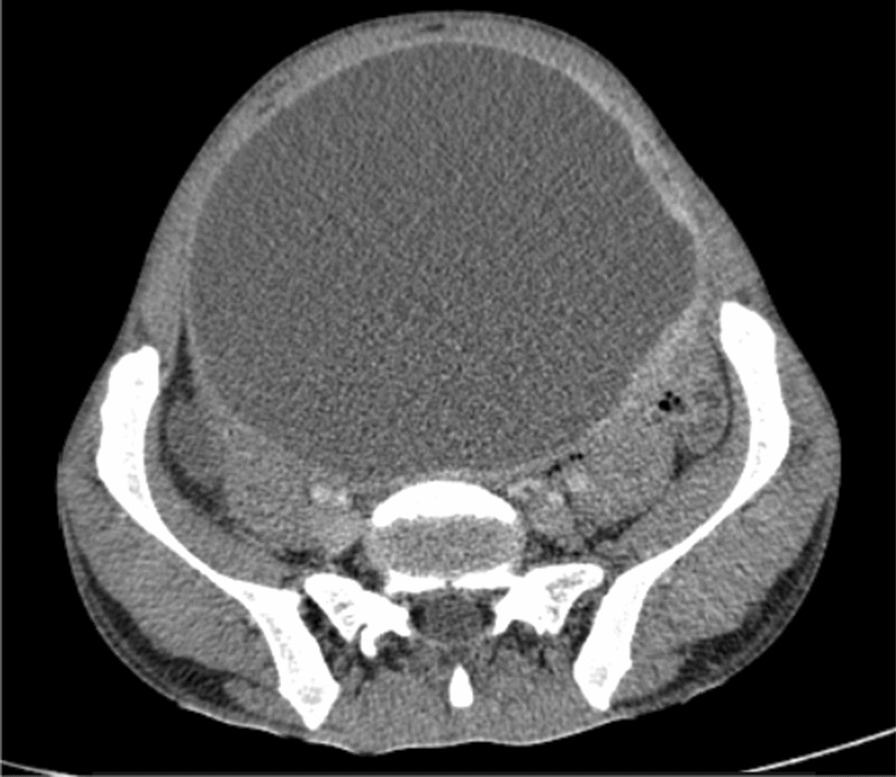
Axial abdominal CT of the patient showing cystic mass
